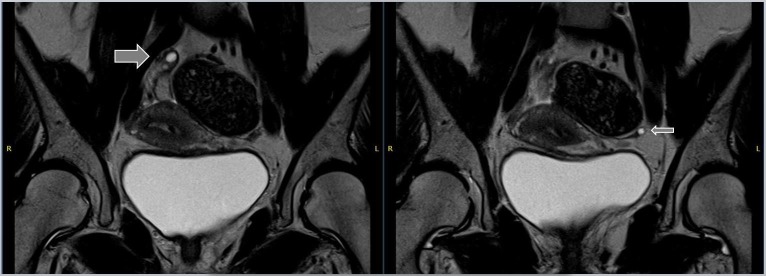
Coronal images from the diagnostic magnetic resonance imaging (MRI) scan Coronal T2-weighted images from the diagnostic MR scan showing the right ovary with three small follicles (thick arrow) with a maximum diameter of 15 mm. The left ovary is not clearly recognizable, but a small cyst is visible with a maximum diameter of 8 mm at the position of the ovary (thin arrow).
